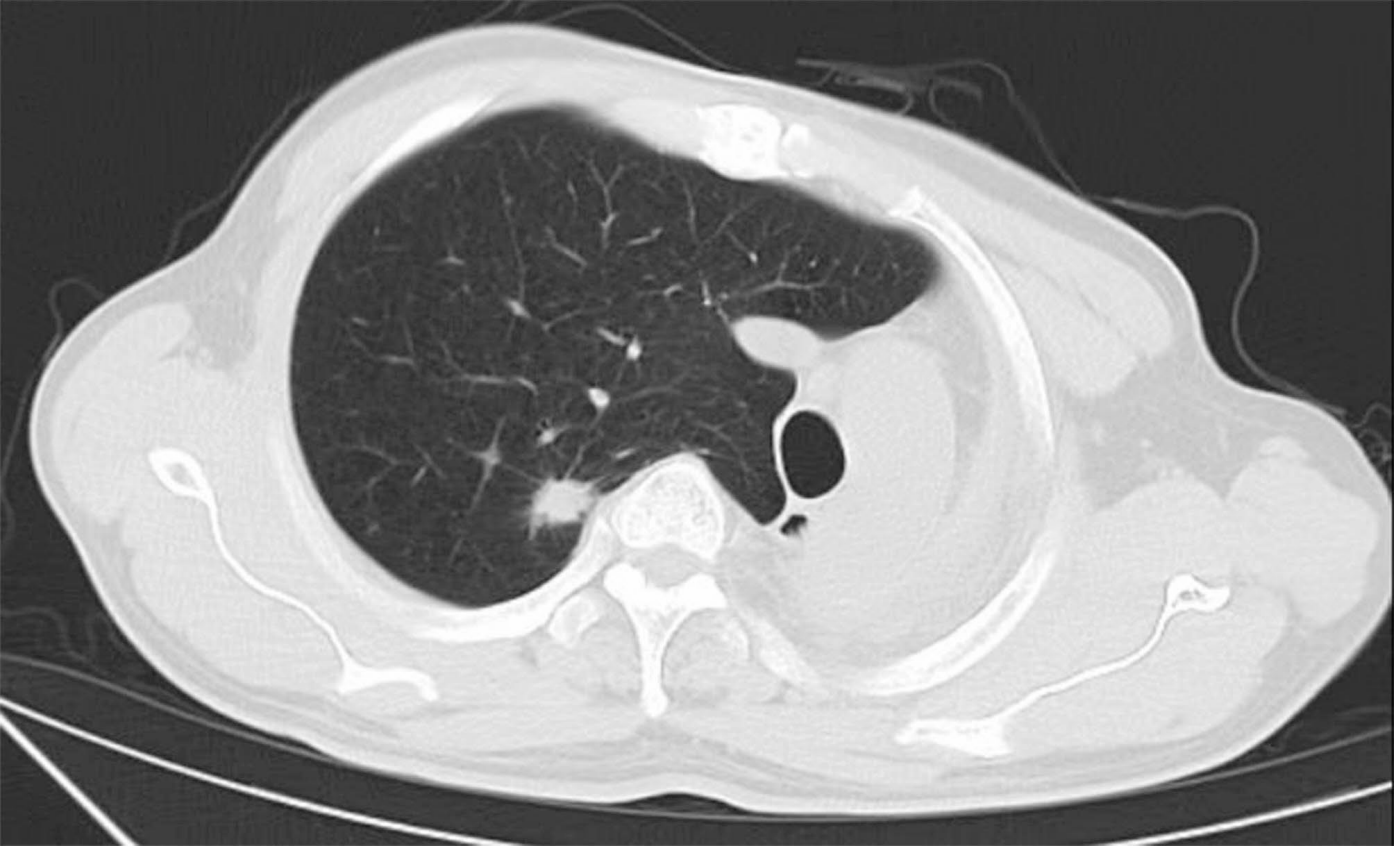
CT revealed a nodule in his right upper lobe
